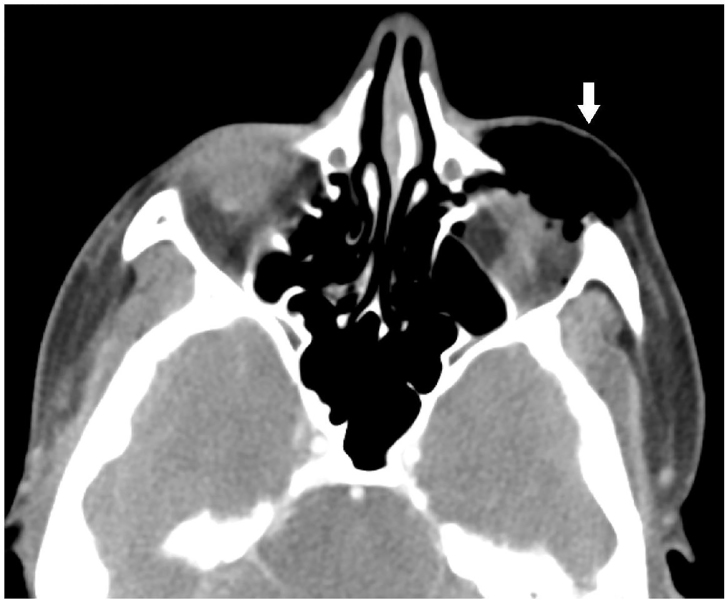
Computed tomography shows subcutaneous emphysema around the left orbit (arrow).
